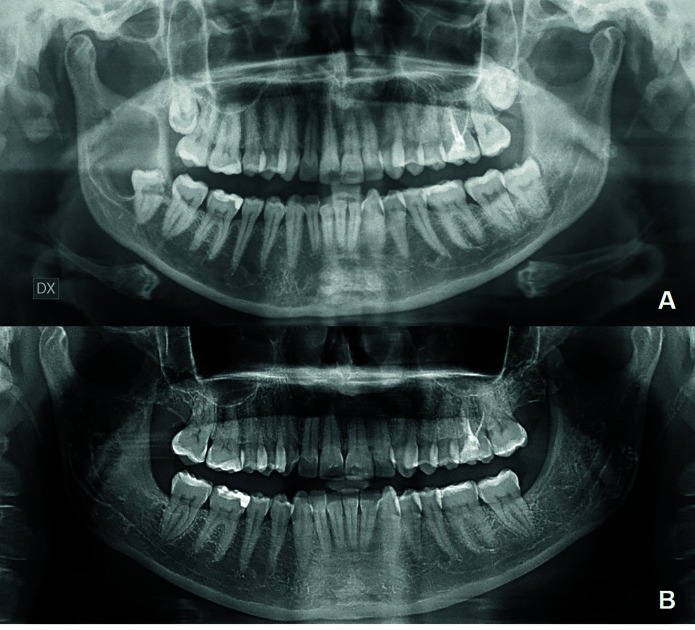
A) Radiographic examination at diagnosis. The lesion includes part of the unerupted 3rd molar and involves IAN with resorption and dislocation; B) Follow-up after 6 months from radical excision.
